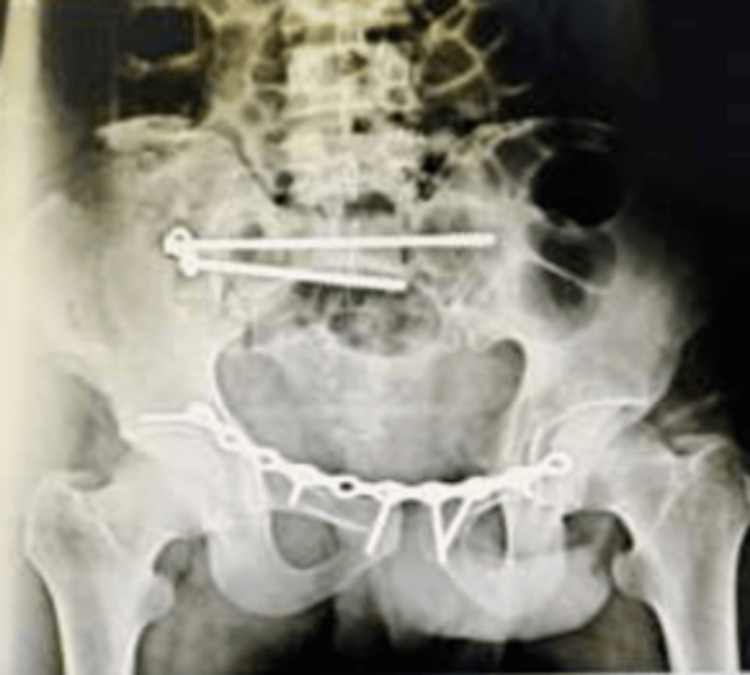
Anteroposterior view of the post-operative X-ray of the patient
